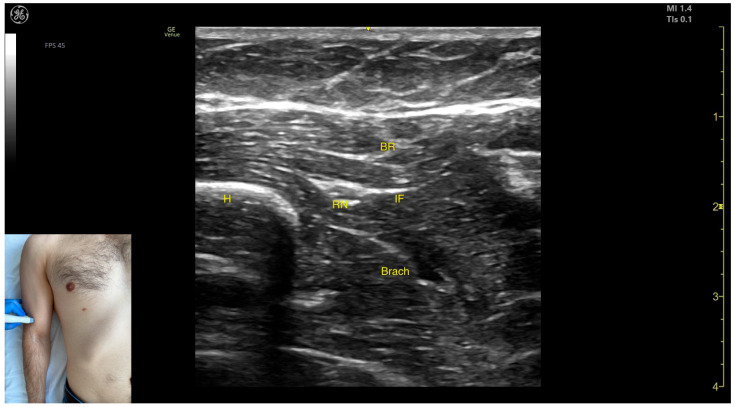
Ultrasound anatomy of the brachioradialis (BR) in the arm compartment with key landmarks: H—humerus; BR—brachioradialis; RN—radial nerve; IF—intermuscular fascia; and Brach—brachialis.
